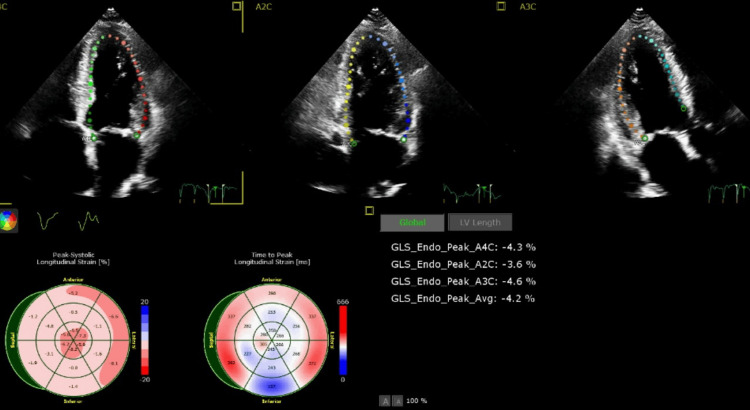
Limited 2D echocardiogram showing decreased global longitudinal strain with relatively better strain (intensely pink area) at the apex compared to other segments 2D: two-dimensional
